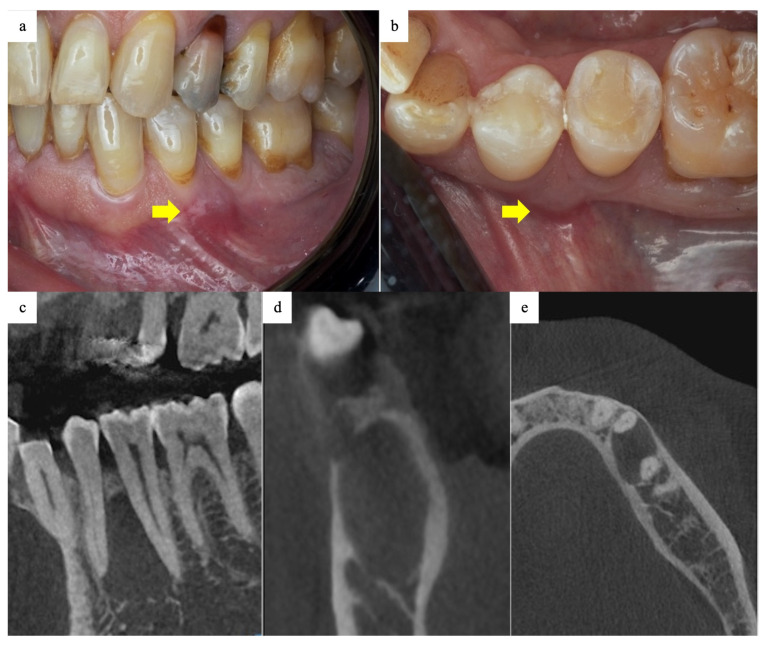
(a,b) Preoperative clinical photographs showing a mild swelling between teeth 35 and 36 (arrow) with normal covering mucosa. (c–e) Preoperative CBCT showing a well-defined radiolucent area related to teeth 35 and 36.
